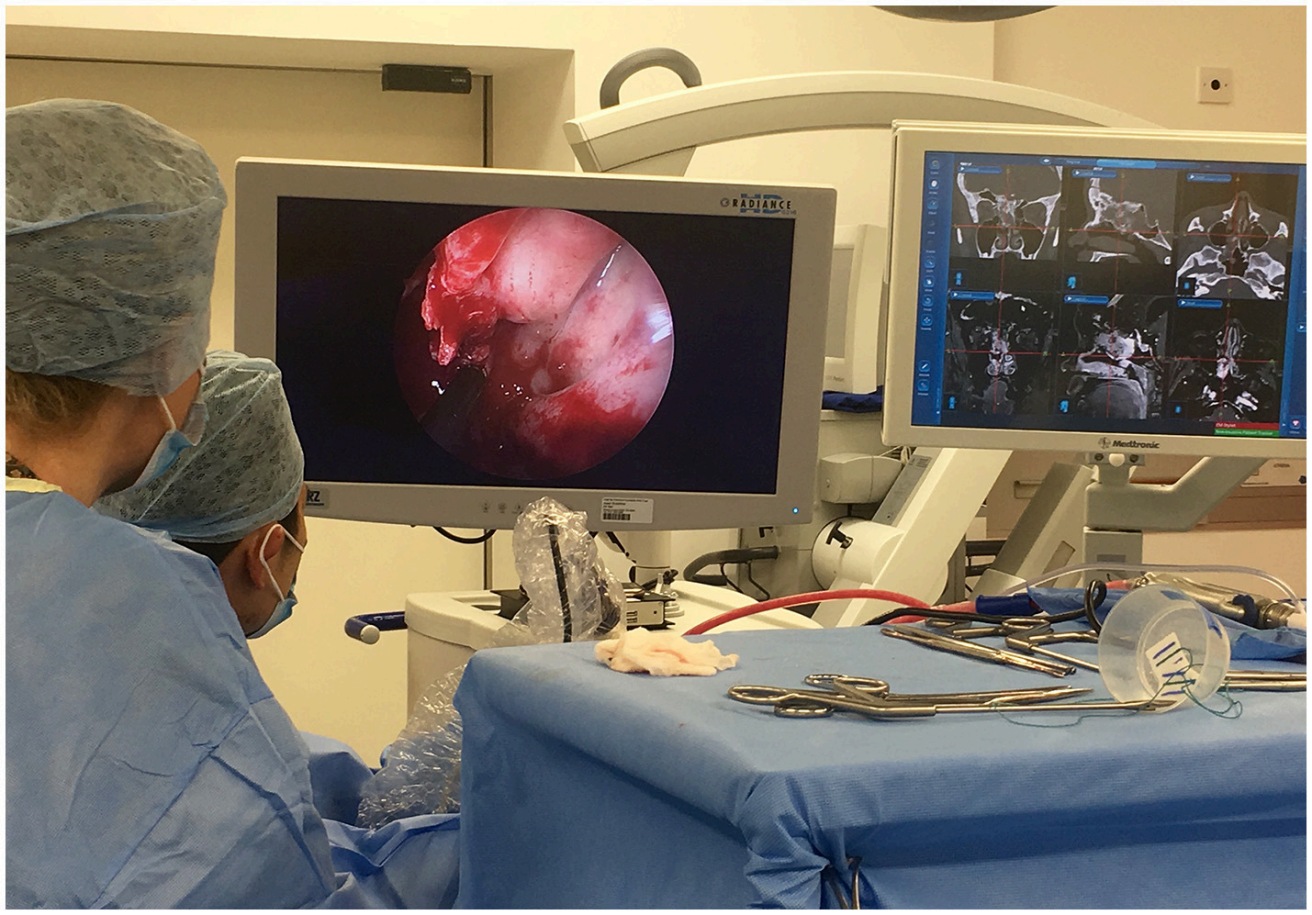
Multimodal use of technology: The use of intraoperative endoscopy for an extended endo-nasal approach, in tandem with neuro-navigation and intraoperative MRI was vital in maximizing resection of this complex recurrent atypical meningioma.
